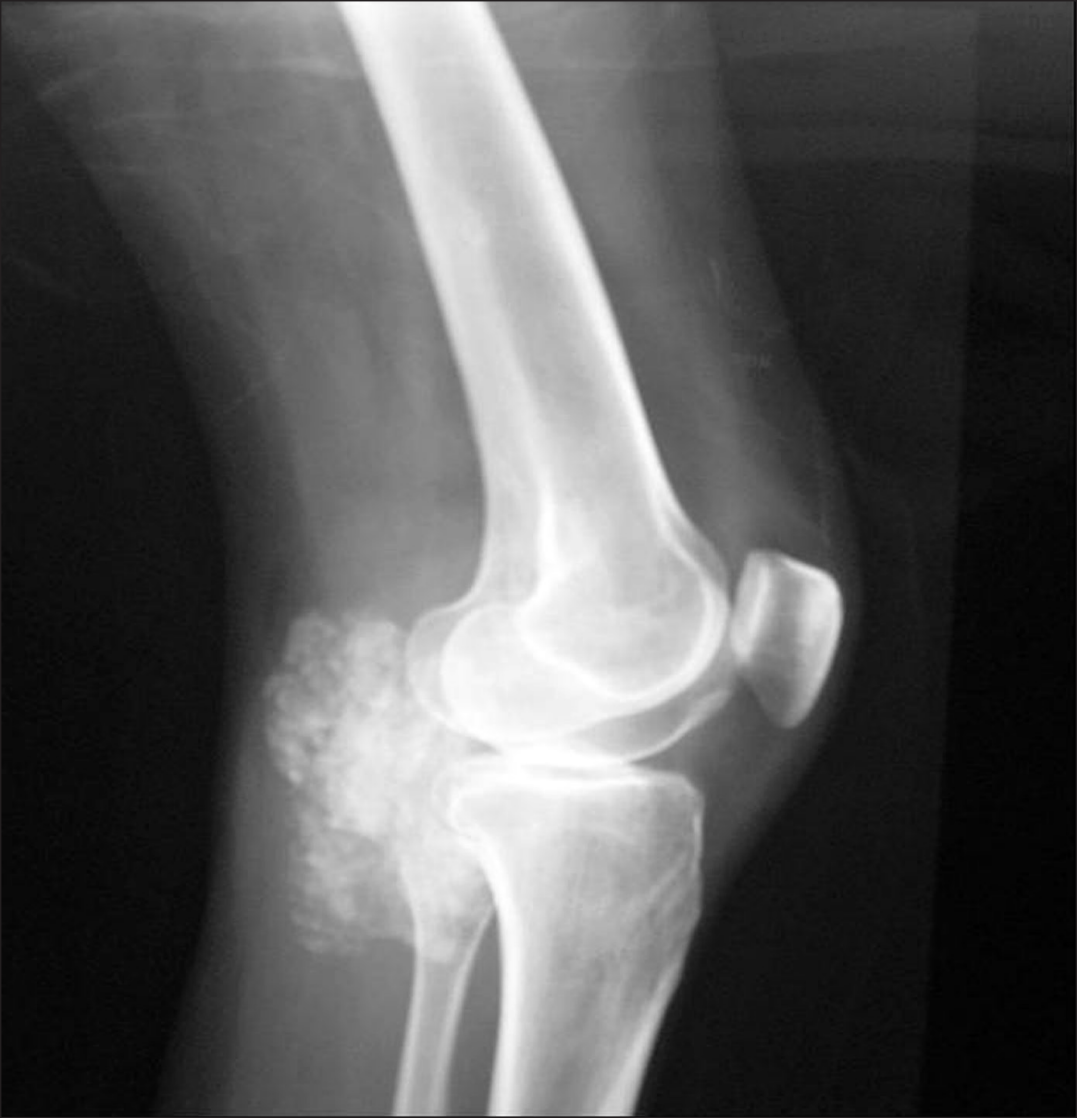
Radiographs of the right knee reveal calcified masses with lucent areas in the popliteal cavity of the knee joint
